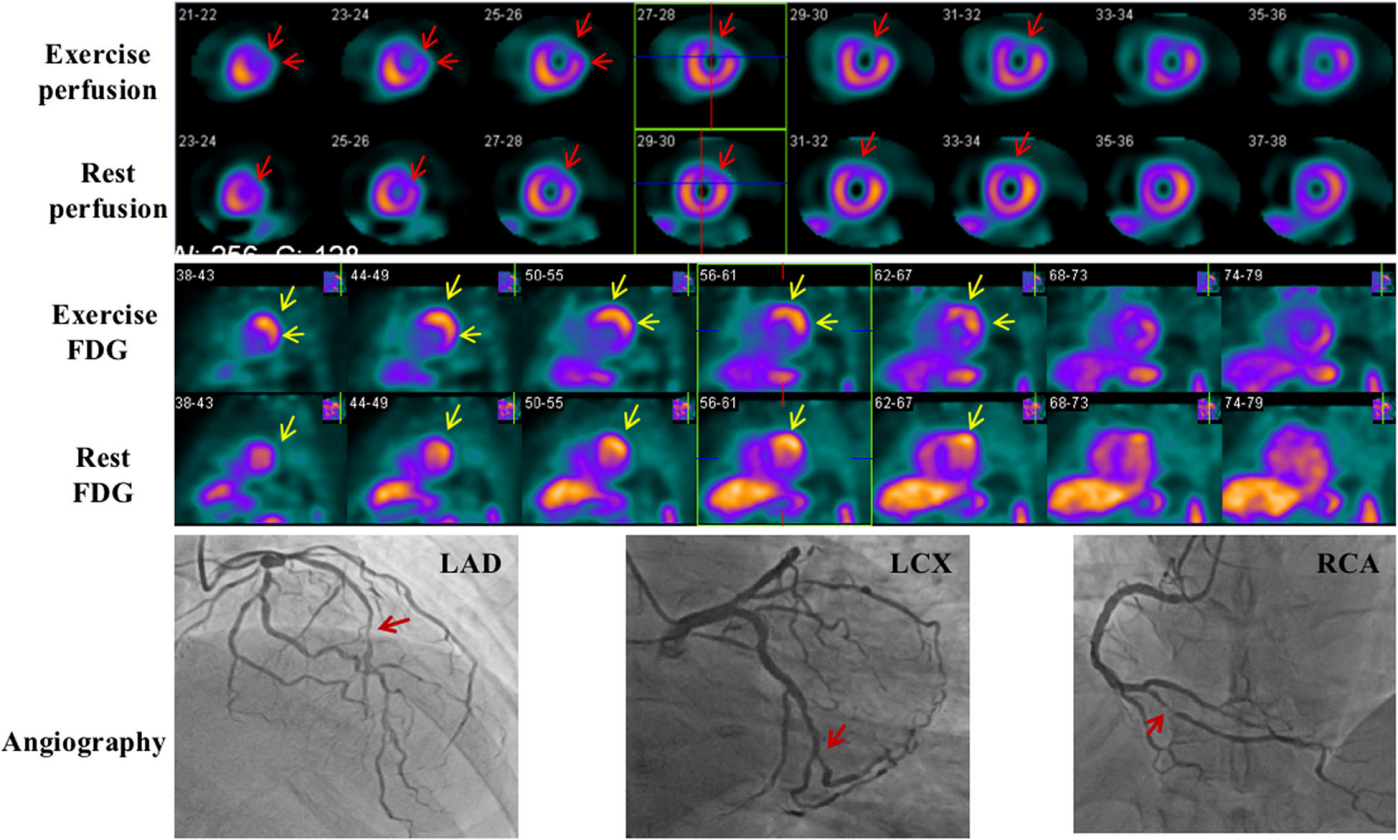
Images of a 55-year-old woman. She had multiple lesions of > 90% stenosis in the LAD, multiple lesions of 60-80% stenosis in the LCX, and a distal lesion of 90% stenosis in the RCA. Perfusion images show partially reversible defects in the anterior and anterolateral wall (‘focal’ pattern, *red arrows*). Resting ^18^F-FDG images show mild uptake in the anterior and anterolateral wall. On exercise images, ^18^F-FDG uptake is further increased compared with resting images, and the involved area is enlarged as well (‘focal’ pattern, *yellow arrows*)
